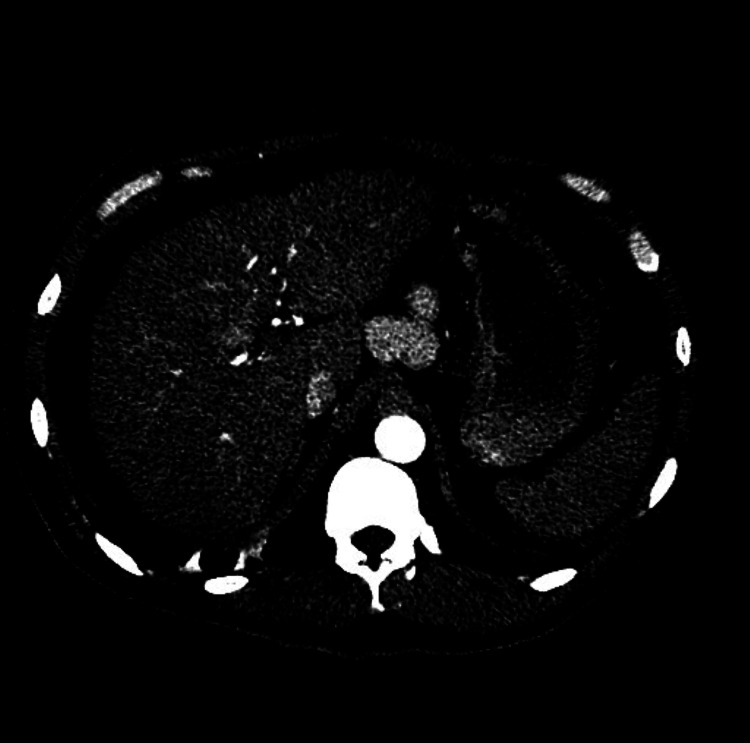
Triple phase CT of the abdomen showing a liver mass in segment VIII with mild arterial enhancement
